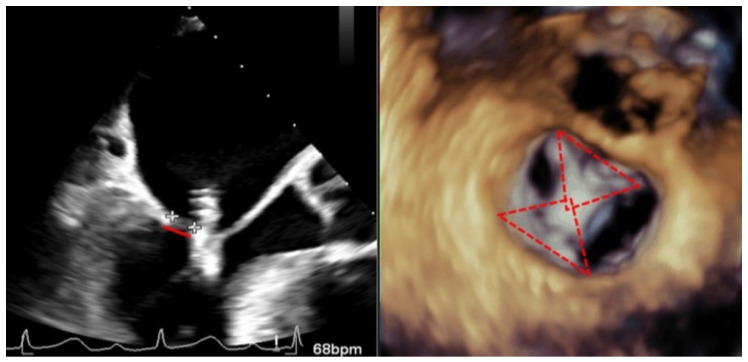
Transesophageal echocardiogram during grasping. (Left) Two-dimensional transesophageal echocardiogram in the view of the left ventricular outflow tract, showing the tension of both leaflets and the measurement of the posterior leaflet introduced in the clip (red line delimited by plus sings). (Right) En-face 3D transesophageal echocardiogram view of the mitral valve with a clip between A2 and P2 with 2 symmetrical pyramids (red dotted lines).
